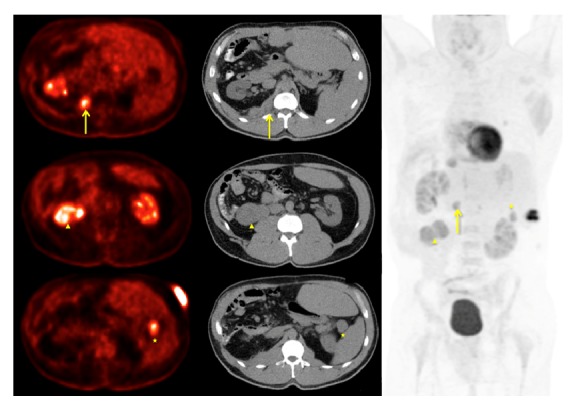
PET scan that demonstrates FDG avidity of omental lymph nodes (stars) and retrocrural lesions (arrows and arrows head).
